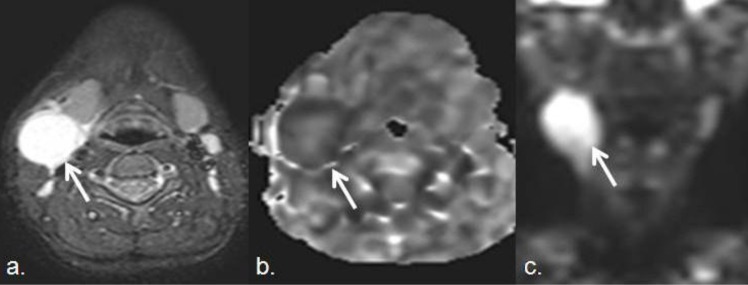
Thirty seven year old female. She had a growing lymph node at left III level, after and oral infection. (a) Transverse STIR shows an enlarged lymph node in the right internal jugular chain (white arrow). (b)The lymph node demonstrates intermediate signal on ADC map (ADC value 2.5 × 10^−3^), and high signal on DWI with high b value (c), due toT2-shine through effect and absence of restricted diffusion. Percutaneous biopsy proved a benign inflammatory origin secondary to an ENT infection.
